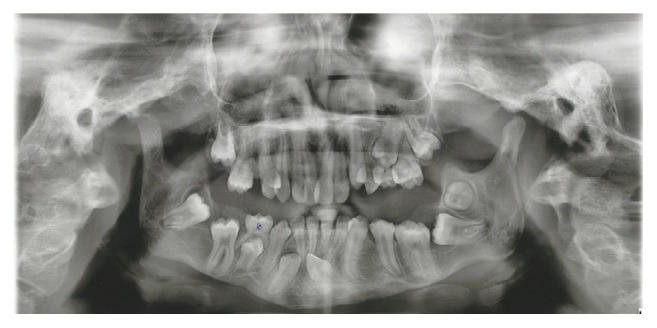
Panoramic radiograph: diverse dental development anomalies, such as maxillary anterior crowding and impacted teeth.
